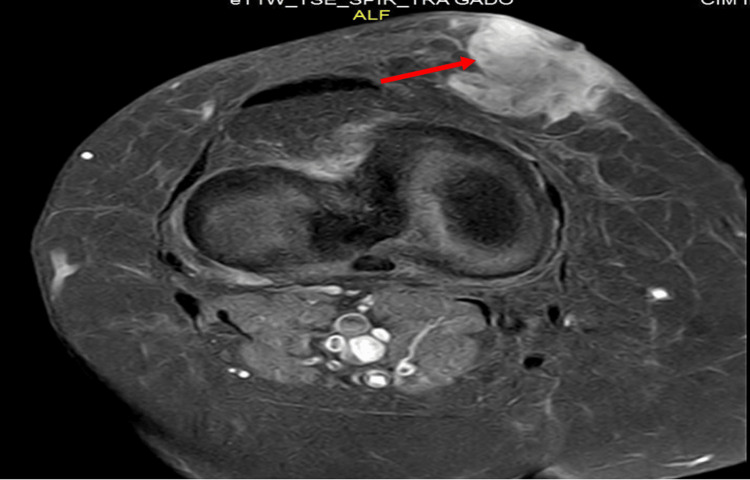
Knee MRI showing a heterogeneously enhancing deep and superficial tissue mass (Red arrow)
